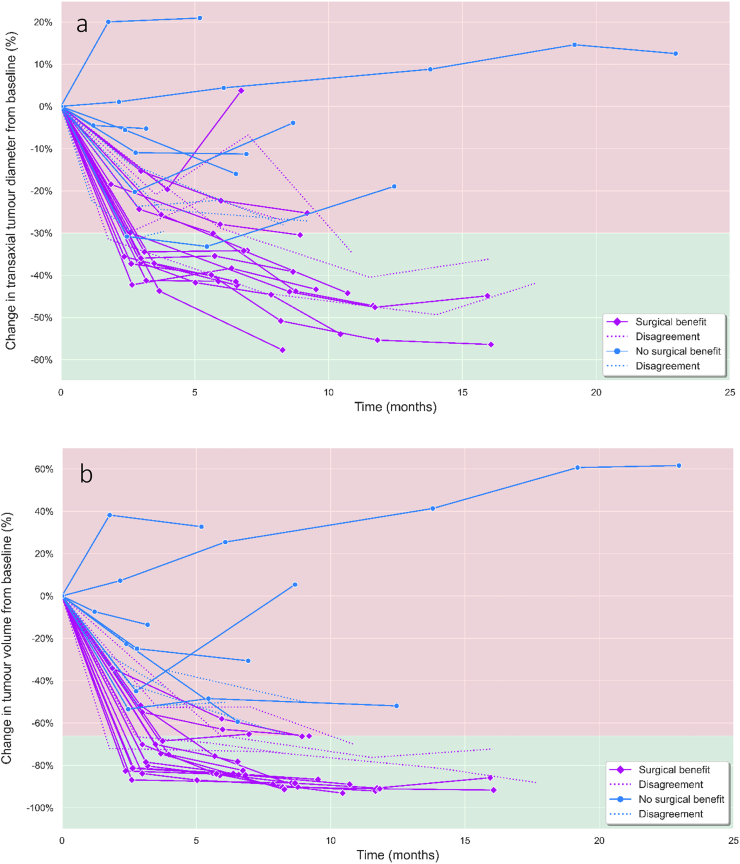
a) Spaghetti plots visualising the individual percentage change in a) transaxial tumour diameter and b) tumour volume over the course of TKI treatment. This analysis was executed on a subset of patients (*n* = 30), who were monitored using additional interim response portal venous CE-CT scans, next to the fixed baseline and preoperative scans. Each line represents a non-metastatic GIST patient, and the colours resemble the concurrent assessment on surgical benefit (magenta = surgical benefit, blue = no surgical benefit). Dashed lines represent patients that surgeons initially disagreed on during surgical benefit assessment. The grids have been divided into two parts by using the predefined thresholds (−30.0 % in diameter and −66.0 % in tumour volume) as presented in current radiological response criteria (green = good response, red = poor response). Tumour progression was observed in three patients with moderate/high risk GISTs of the ileum and stomach, all harbouring KIT exon 11 mutations. One of these patients was treated for 23.1 months, since they initially refrained from surgical resection. (For interpretation of the references to colour in this figure legend, the reader is referred to the web version of this article.)
